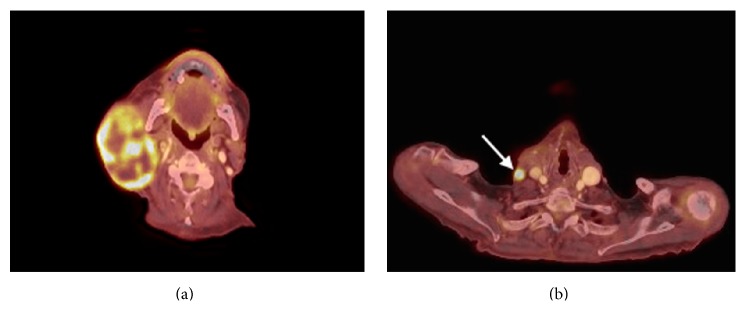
PET: axial images demonstrating FDG avidity in right parotid mass and extension to right subclavian vein.
